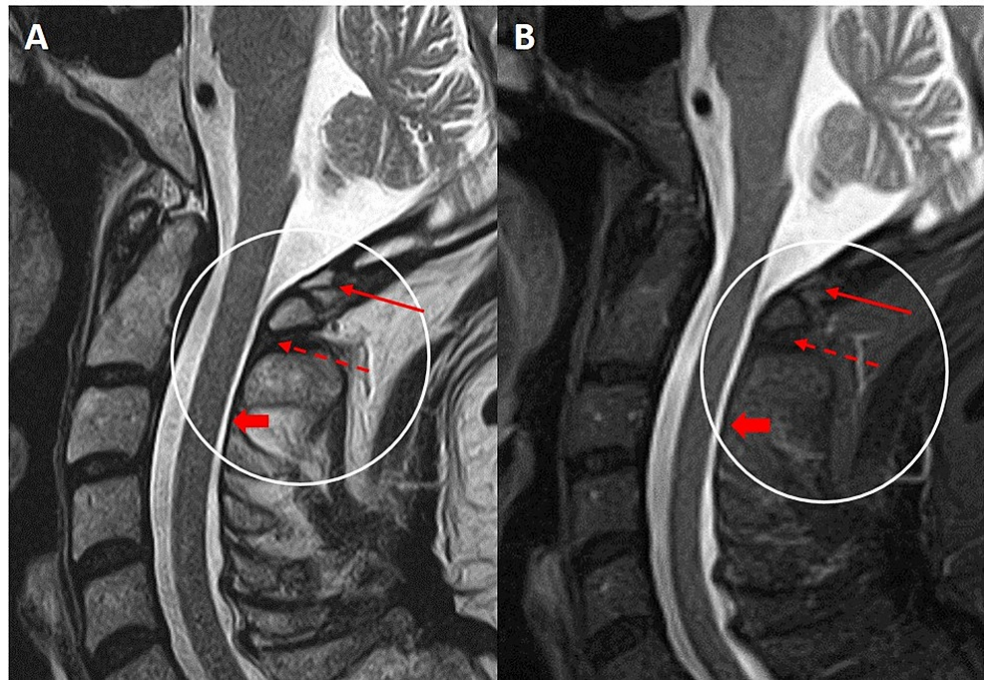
Sagittal T2 weighted (A) and STIR (B) Cervical MRI sequences demonstrating the normal imaging appearance of the posterior atlanto-occipital membrane (red arrow) extending between the opisthion of the occiput and posterior C1 arch. A small area of ossification is present within the membrane. The posterior atlanto-axial membrane (red dashed arrow) extends between the posterior C1 arch and C2 lamina where it is continuous with the ligamentum flavum inferior to C2 (red block arrow). The two membranes are normally indistinguishable from the posterior dura at C1-C2 and together comprise the posterior atlanto-occipital membrane complex. STIR: short tau inversion recovery, MRI: magnetic resonance imaging
